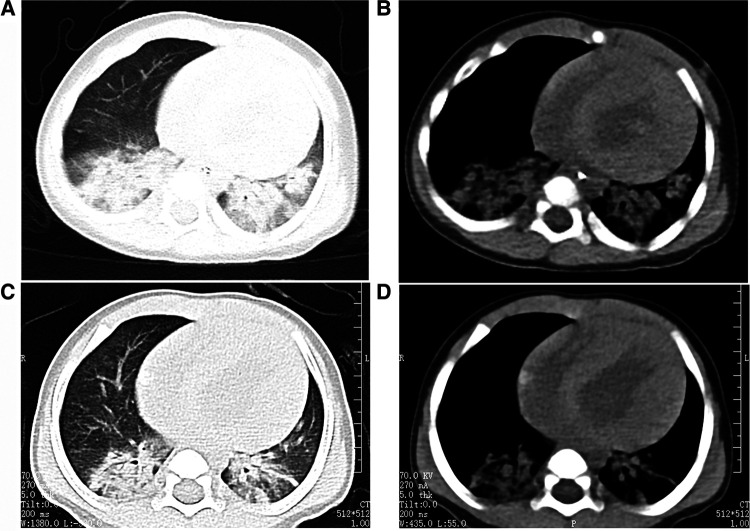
The child's chest CT scans indicated bilateral pneumonia. (A and B: before treatment, C and D: after treatment), with a significantly enlarged cardiac silhouette. After one week of treatment, the pulmonary imaging showed improvement, but there was no noticeable change in the size of the cardiac silhouette.
